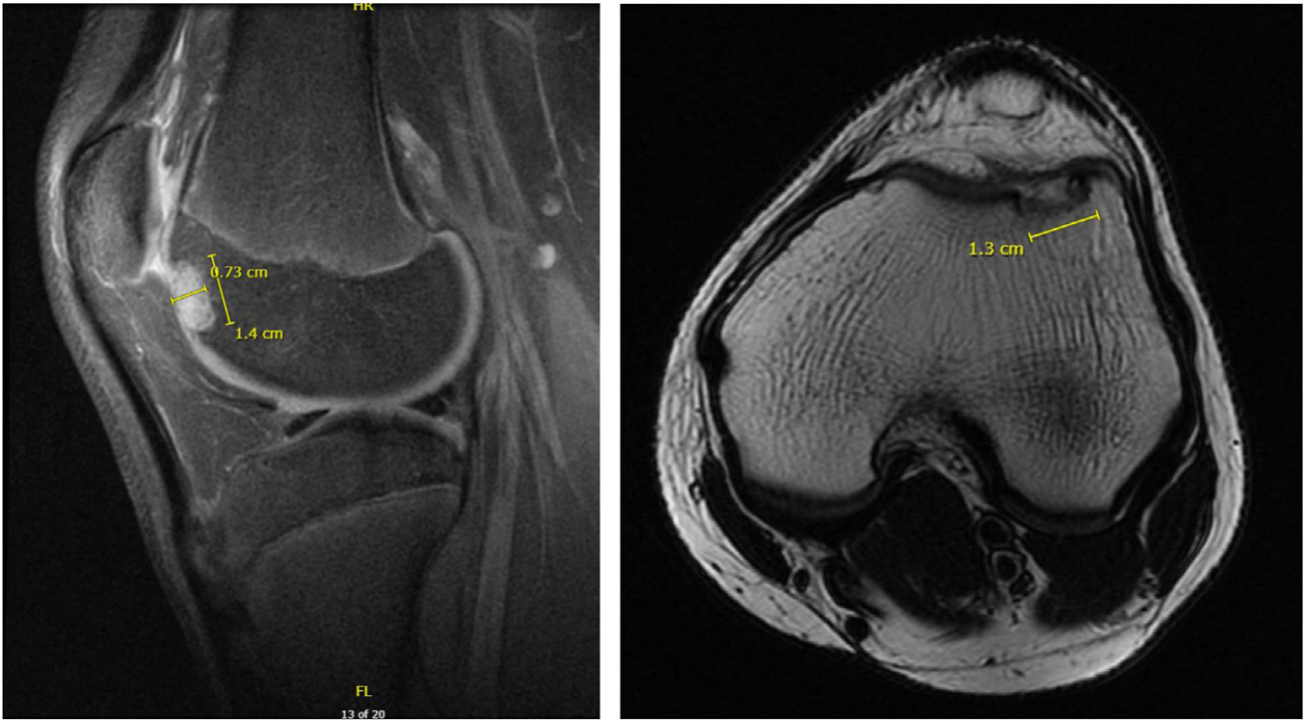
Magnetic resonance imaging scans of left knee in 16-year-old male patient showing lateral trochlea osteochondral defect measuring roughly 14 mm × 13 mm and involving subchondral bone.
